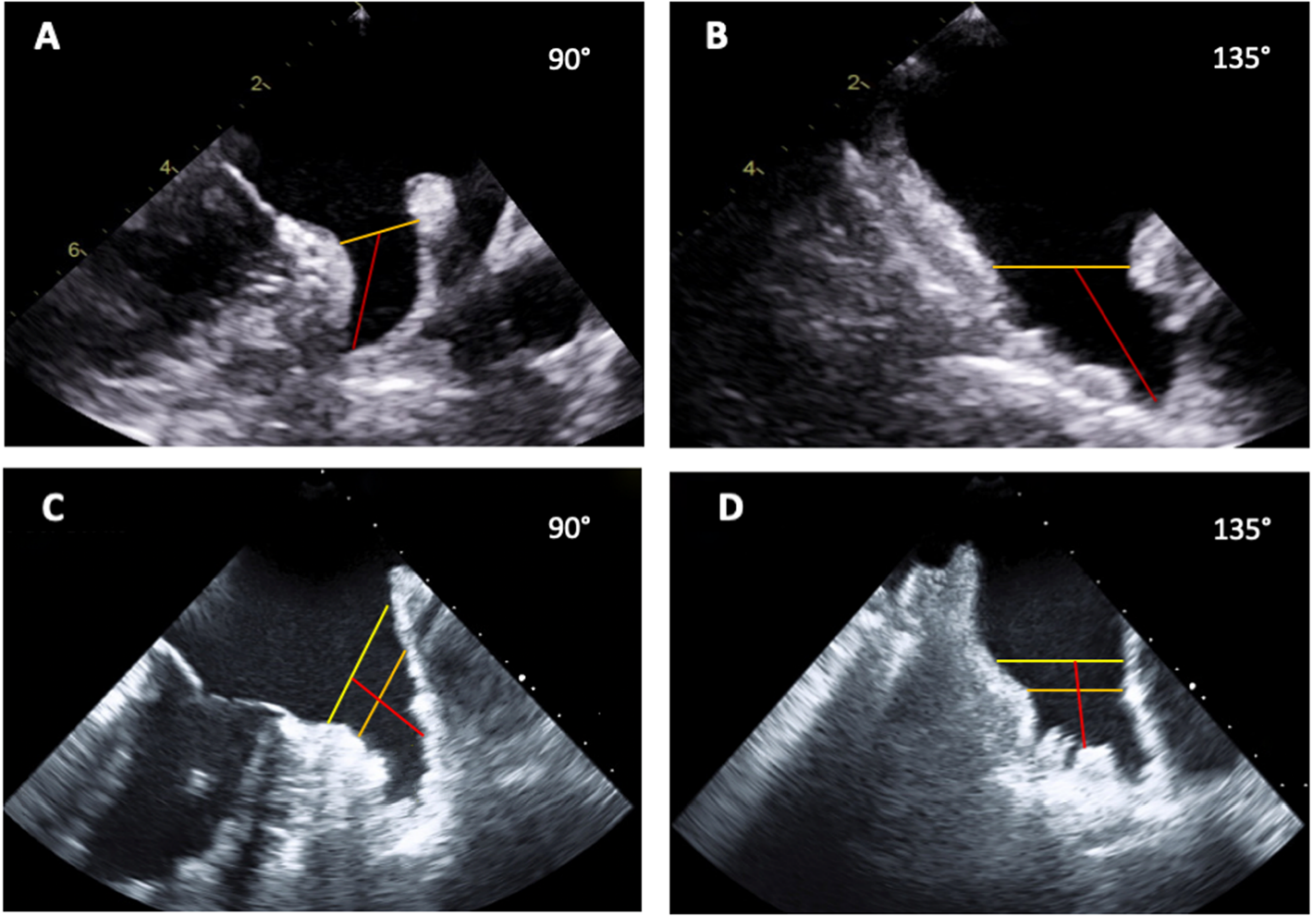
Transesophageal echocardiography measurements for left atrial appendage closure using the Watchman device and the the Amplatzer Cardiac Plug/Ultraseal devices. For the Watchman device (a/b), the LZ (orange line) measurement is taken from the circumflex artery medially to 1-2 cm laterally inside the limbus, and the depth (red line) is measured from the LZ to the most distal tip of the main lobe. For the Amplatzer or the Ultraseal devices (c/d), the orifice (yellow line) is the line that connects the circumflex artery to the pulmonary vein ridge, and the LZ (orange line) is measured at 10-12 mm within the LAA orifice .The depth (red line) is measured from the orifice to the back wall of the LAA in a perpendicular line. LAA: left atrial appendage; LZ: landing zone
